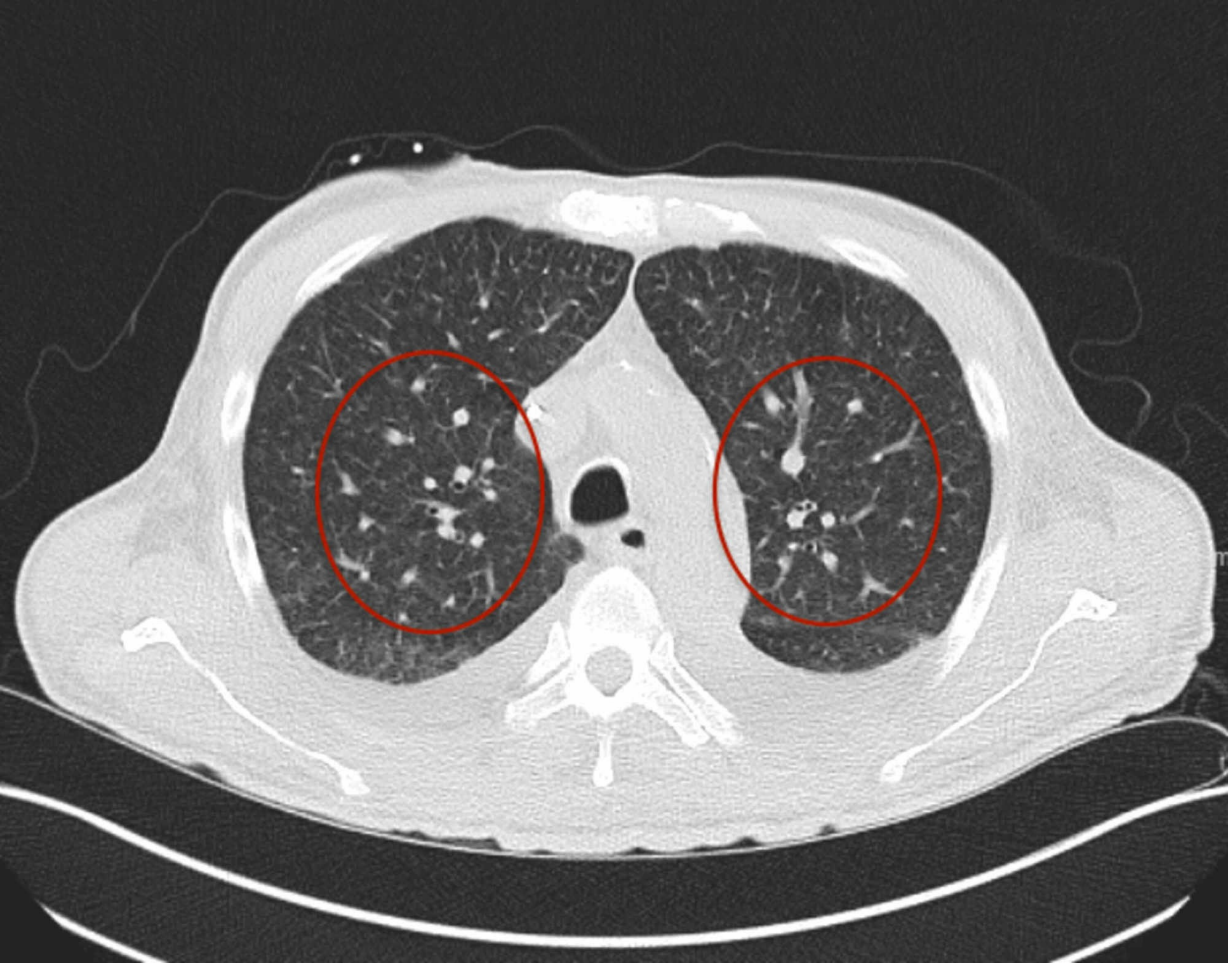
CT of the chest showing small miliary nodules in the lungs consistent with septic emboli
